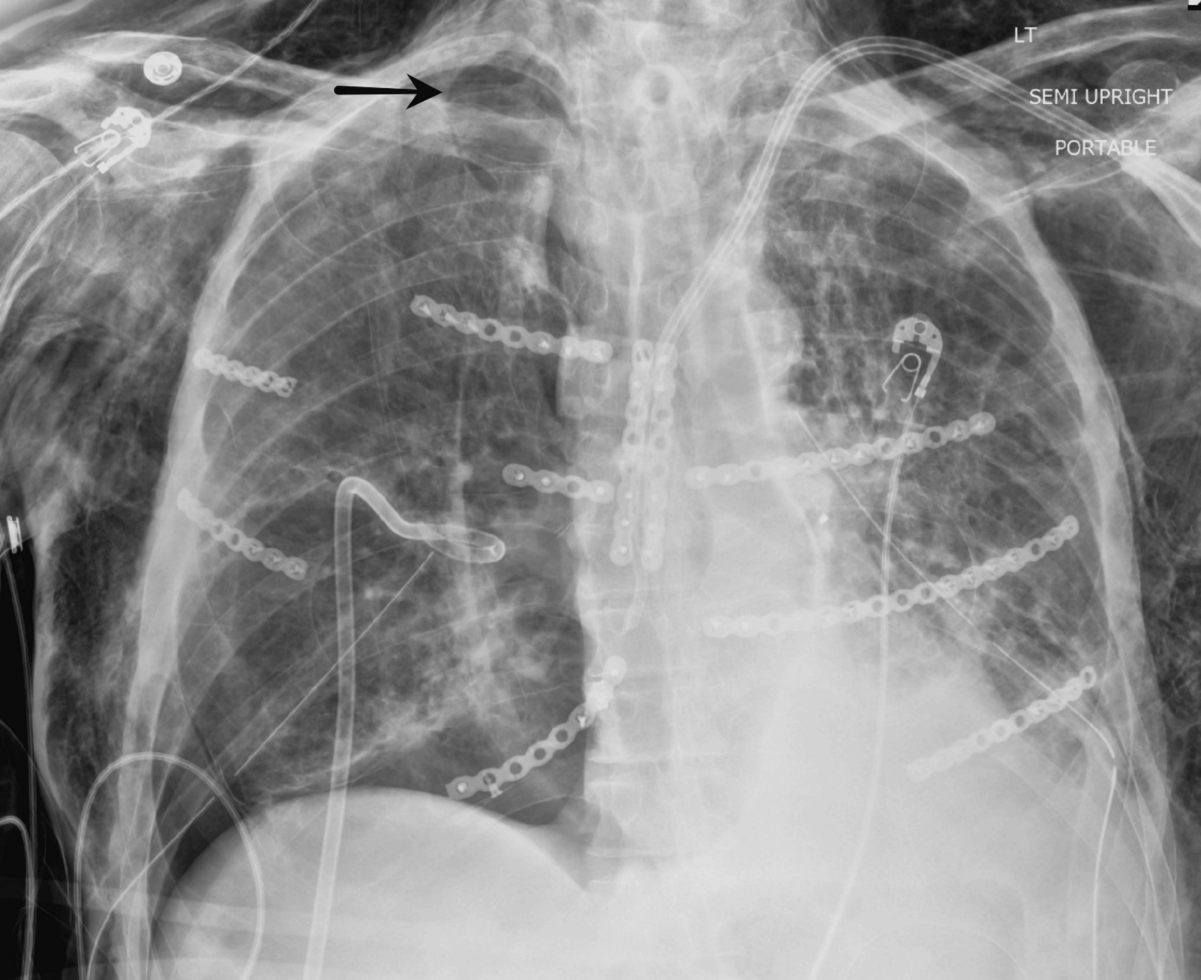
A chest X-ray with right apical pneumothorax (black arrow)
